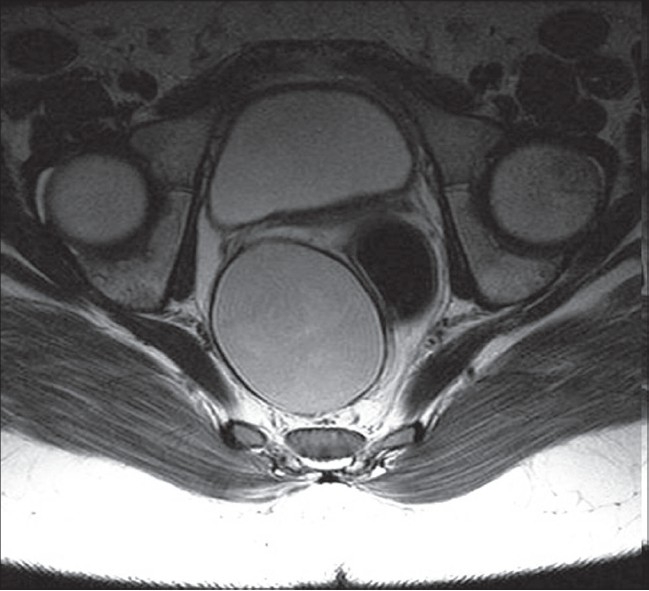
The pelvic T1-weighted magnetic resonance imaging in the transversal slice showing a presacral mass with cystic content
